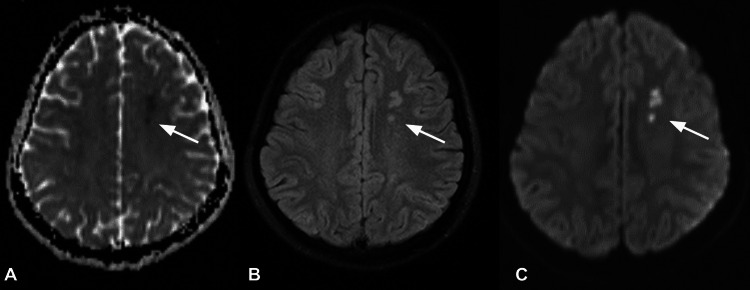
ADC (A), FLAIR (B) and DWI (C) axial sequence in MRI brain showing multifocal acute watershed infarction in left centrum semiovale region (white arrow) ADC: Apparent Diffusion Coefficient; DWI: Diffusion-Weighted Imaging; FLAIR: Fluid Attenuated Inversion Recovery; MRI: Magnetic Resonance Imaging
